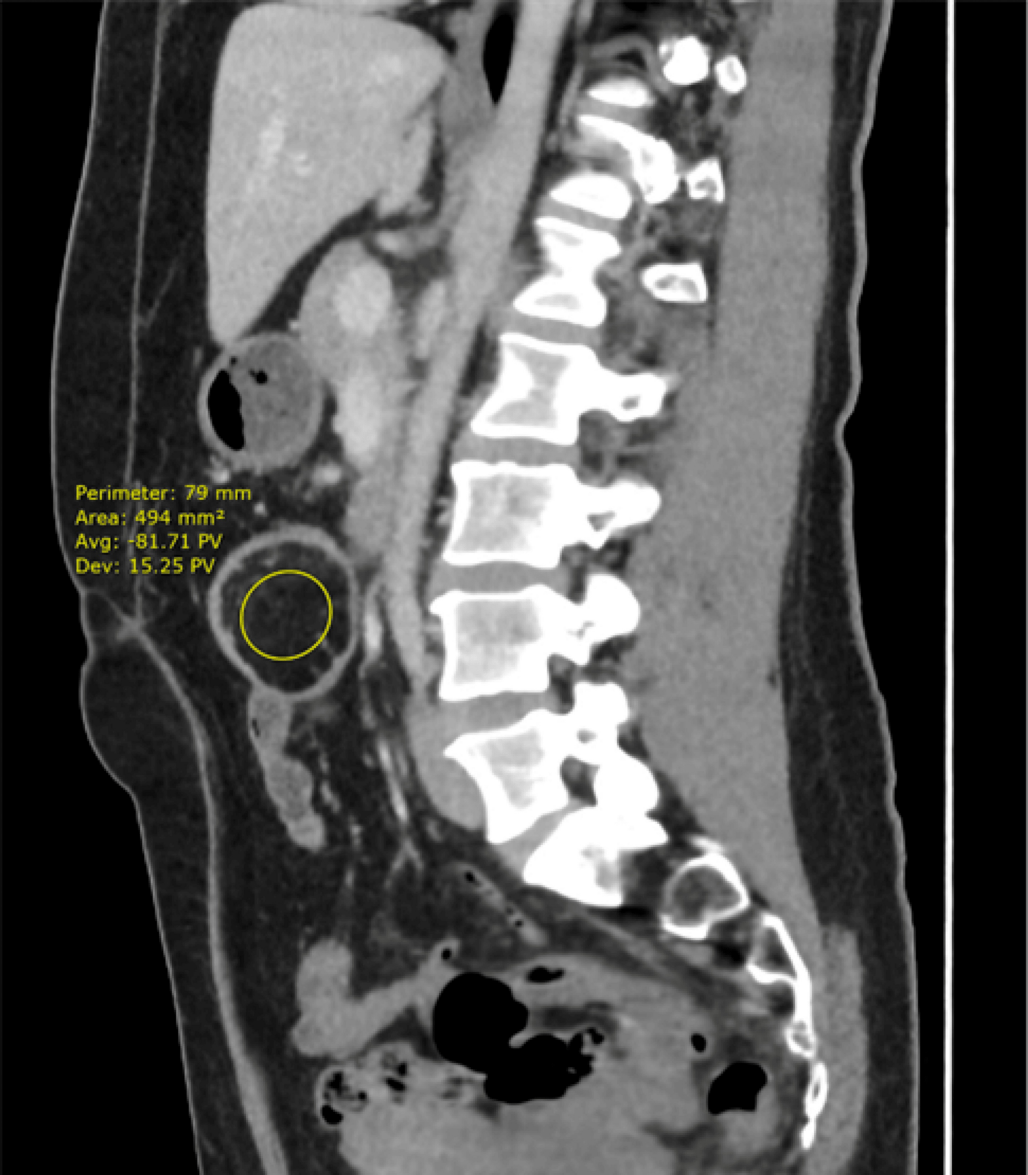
Sagittal view of the CT scan in the portal venous phase showing lipoma as the lead point for colo-colic intussusception HU: Hounsfield Unit The average HU density of the lipoma is shown as -81.
